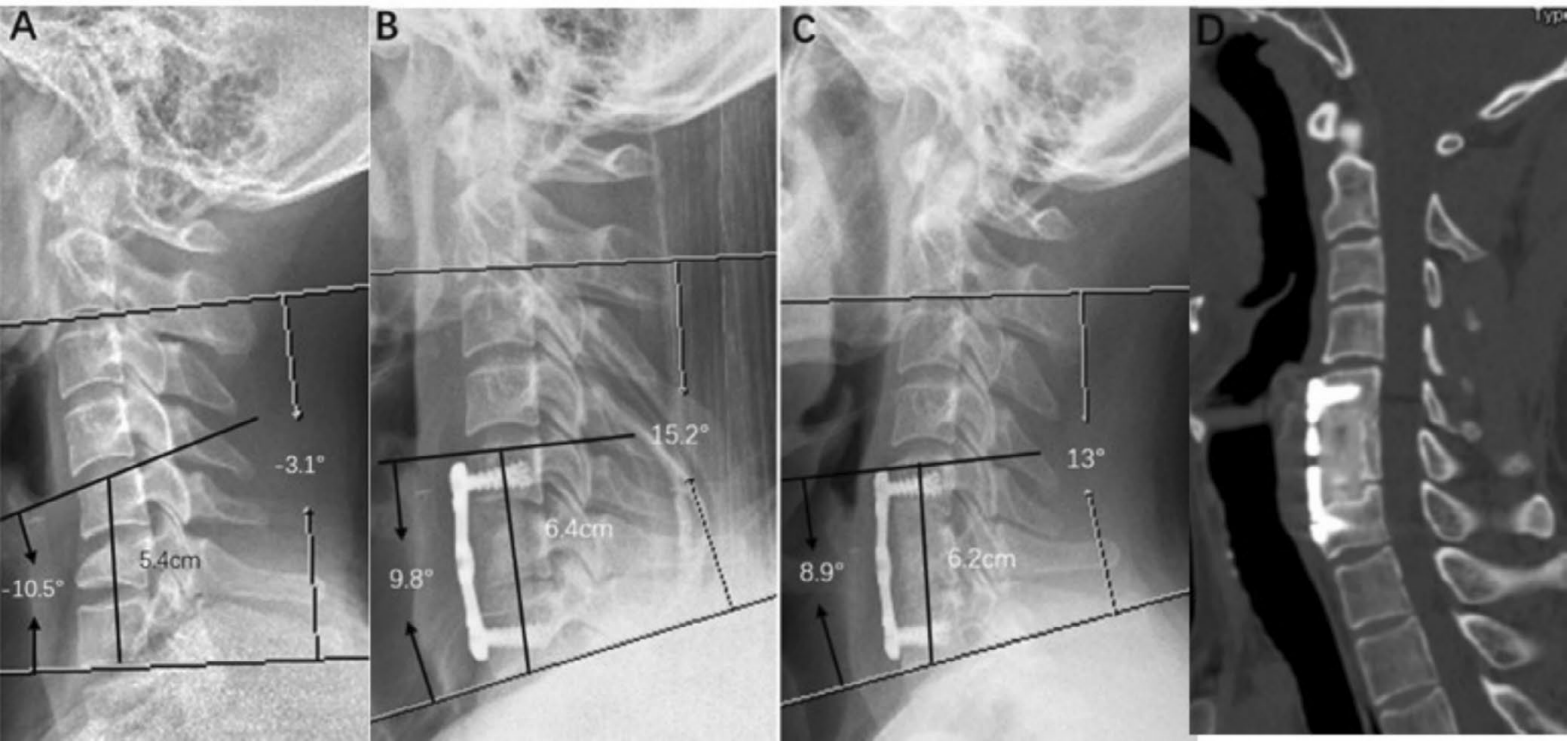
A 40‐year‐old man who underwent 1‐level corpectomy with a nano‐hydroxyapatite/polyamide66 strut for cervical reconstruction. The preoperative cervical X‐ray (A) (a significant collapse of the C6 vertebra): 1 week postoperatively lateral X‐ray (B) (C6 corpectomy and the n‐ha/pa66 cage used for reconstruction improved CL, SA, and IH). The radiographic films (C) and 3D‐CT (D) scan showing satisfying bony fusion with a little cage subsidence at the 9.5‐year follow‐up.
